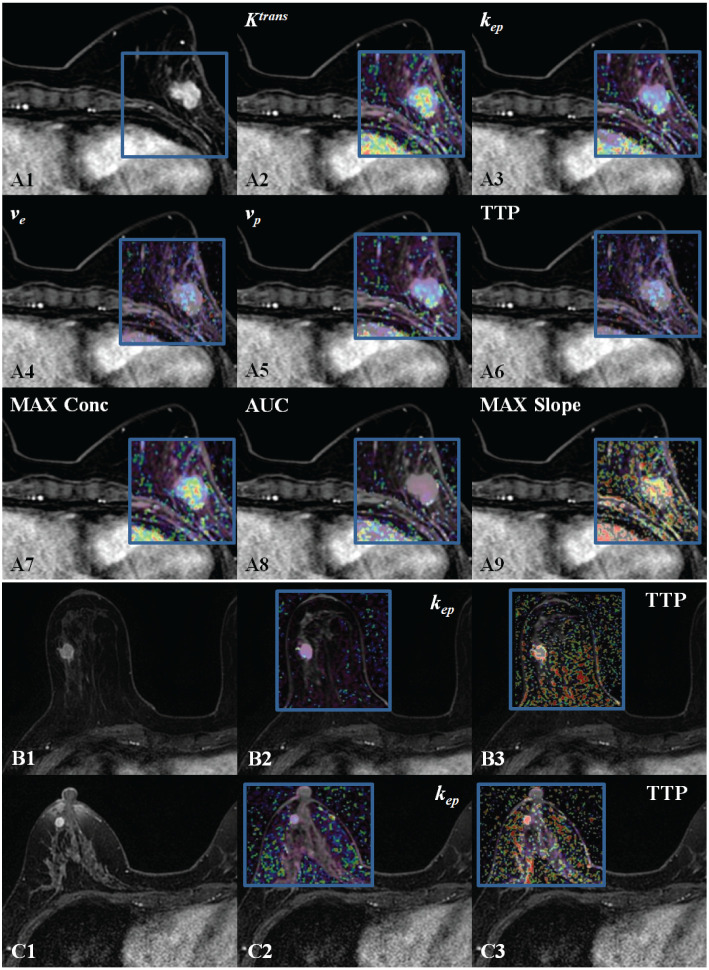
DISCO CE-MRI original map and pseudo color map generated by the tumor area. Clearly enhanced lesions can be seen within the gland of the breasts. (A1), female with breast cancer (HER 2+). (A2–A9) are the pseudo color images of *K^trans^
